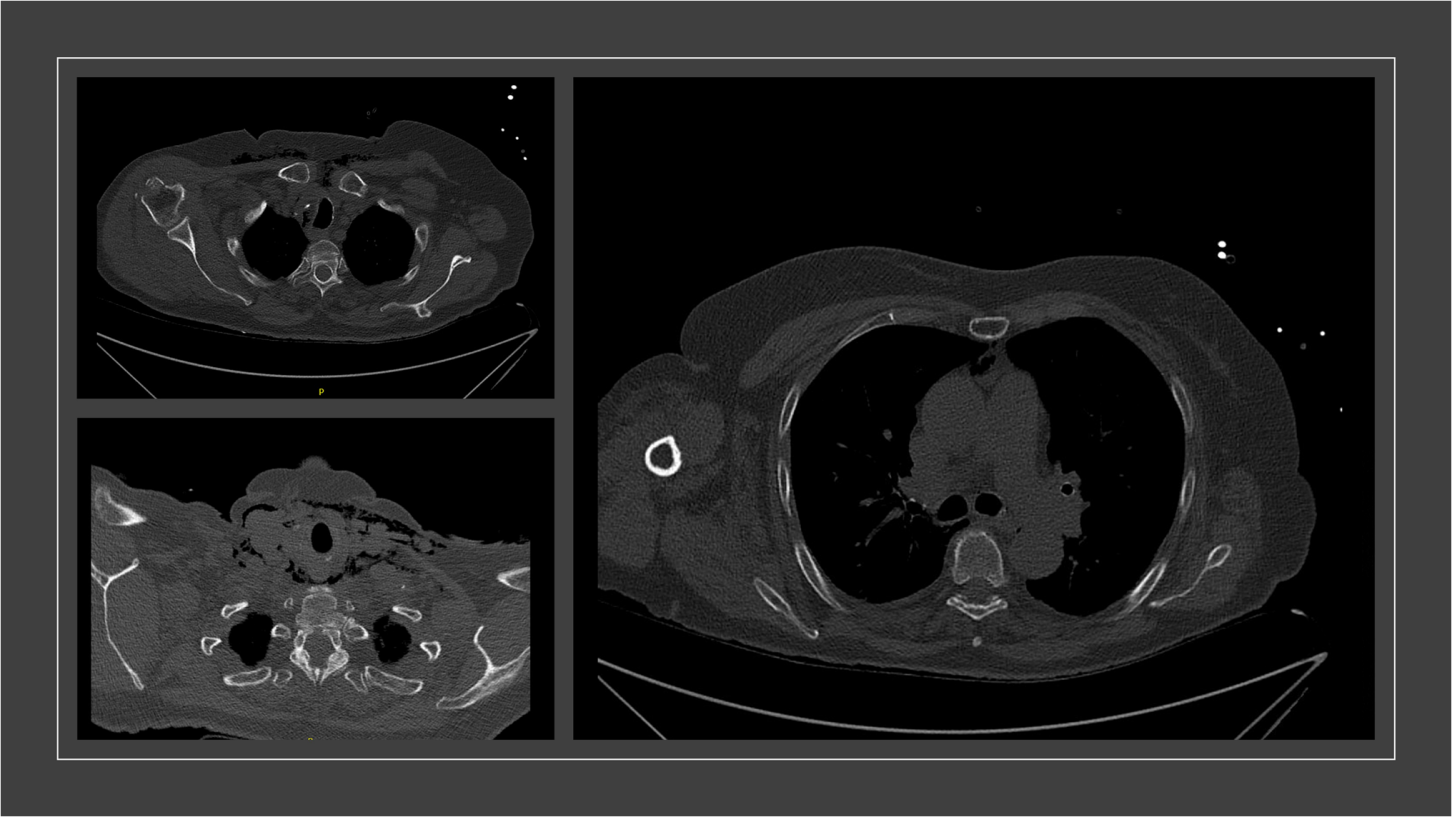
Following computed tomography of the thorax was able to diagnose further spread of emphysema. It extended from the neck to retrosternal and into the mediastinum
